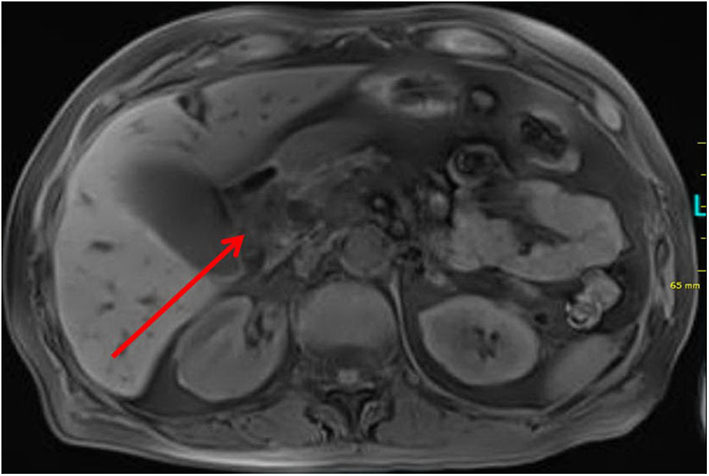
MRI scan showing the tumor in pancreatic region in axial projection.
